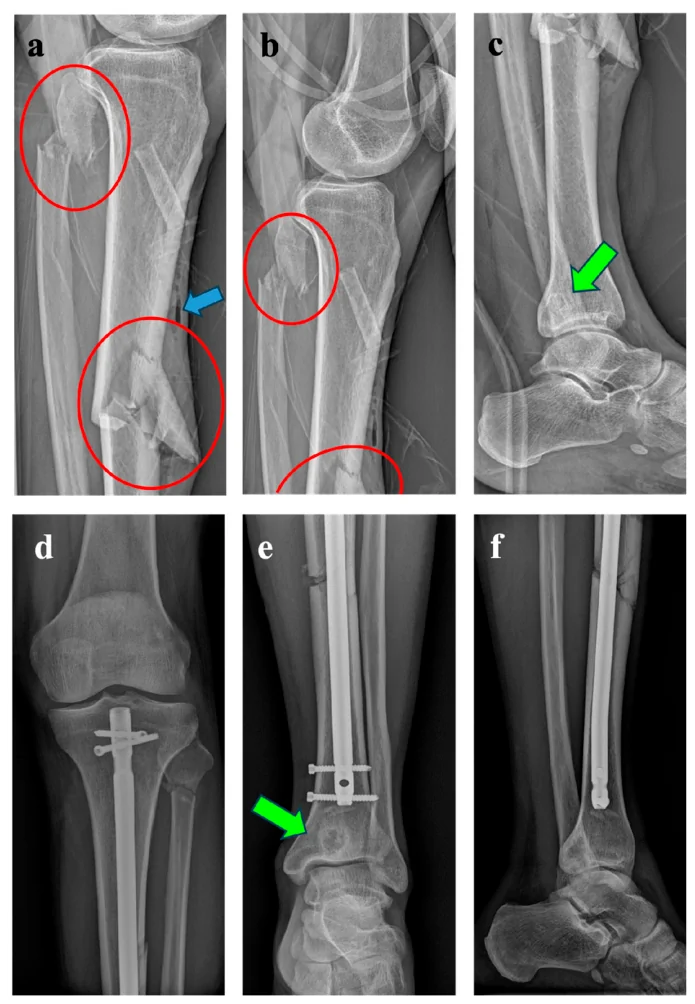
Preoperative radiographic images (a–c) demonstrating a proximal fibular diaphyseal fracture and a mid-diaphyseal fracture of the tibia, highlighted by red circles. Postoperative radiographs (d–f) following external reduction and intramedullary nail fixation. The blue arrow indicates the site of exposure in (a) (Gustilo type I). The green arrow highlights an osteolytic lesion (Grade IA Lodwick and Madewell), visible on both preoperative (c) and postoperative (e) radiographic images.
